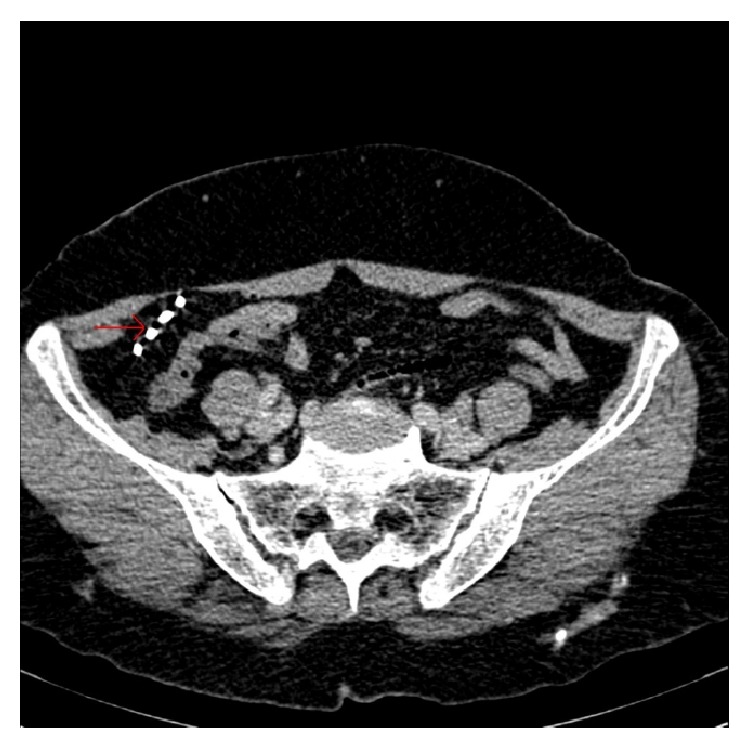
CT scan shows the intrauterine device in the right upper front part of the abdomen.
